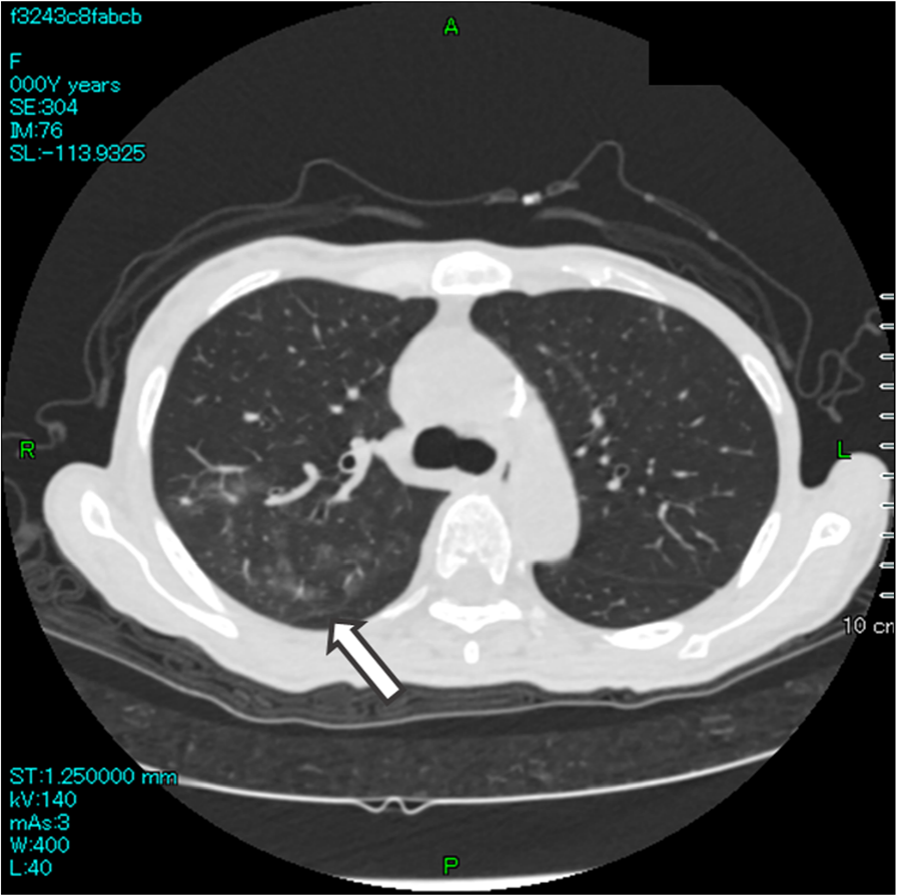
Computed tomography (CT) images of the lung field of Case 5. CT revealed ground-glass opacity and granular shadows in the right upper lobe of the lung field (arrow)
